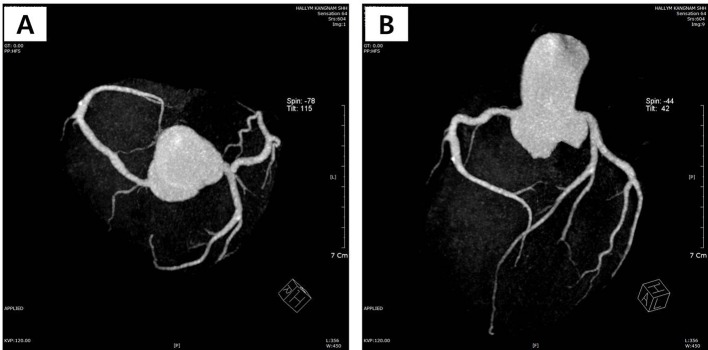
(A, B) Coronary computed tomography showing normal coronary arteries without stenosis.
